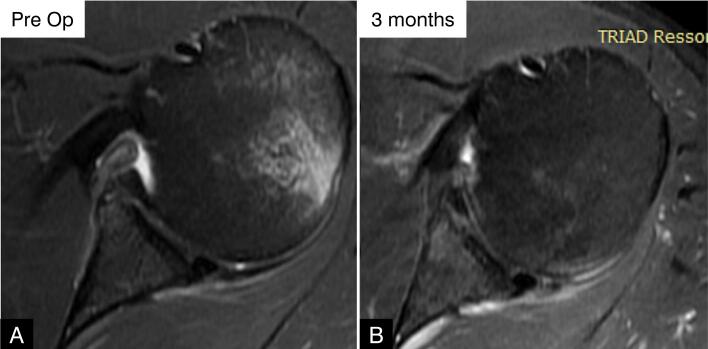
Axial MRI scan of the right shoulder. (A) Preoperative image showing a detached, incomplete labrum and (B) 3 months postoperatively showing an intact but heterogeneous labrum (grade II linear hypersignal).
